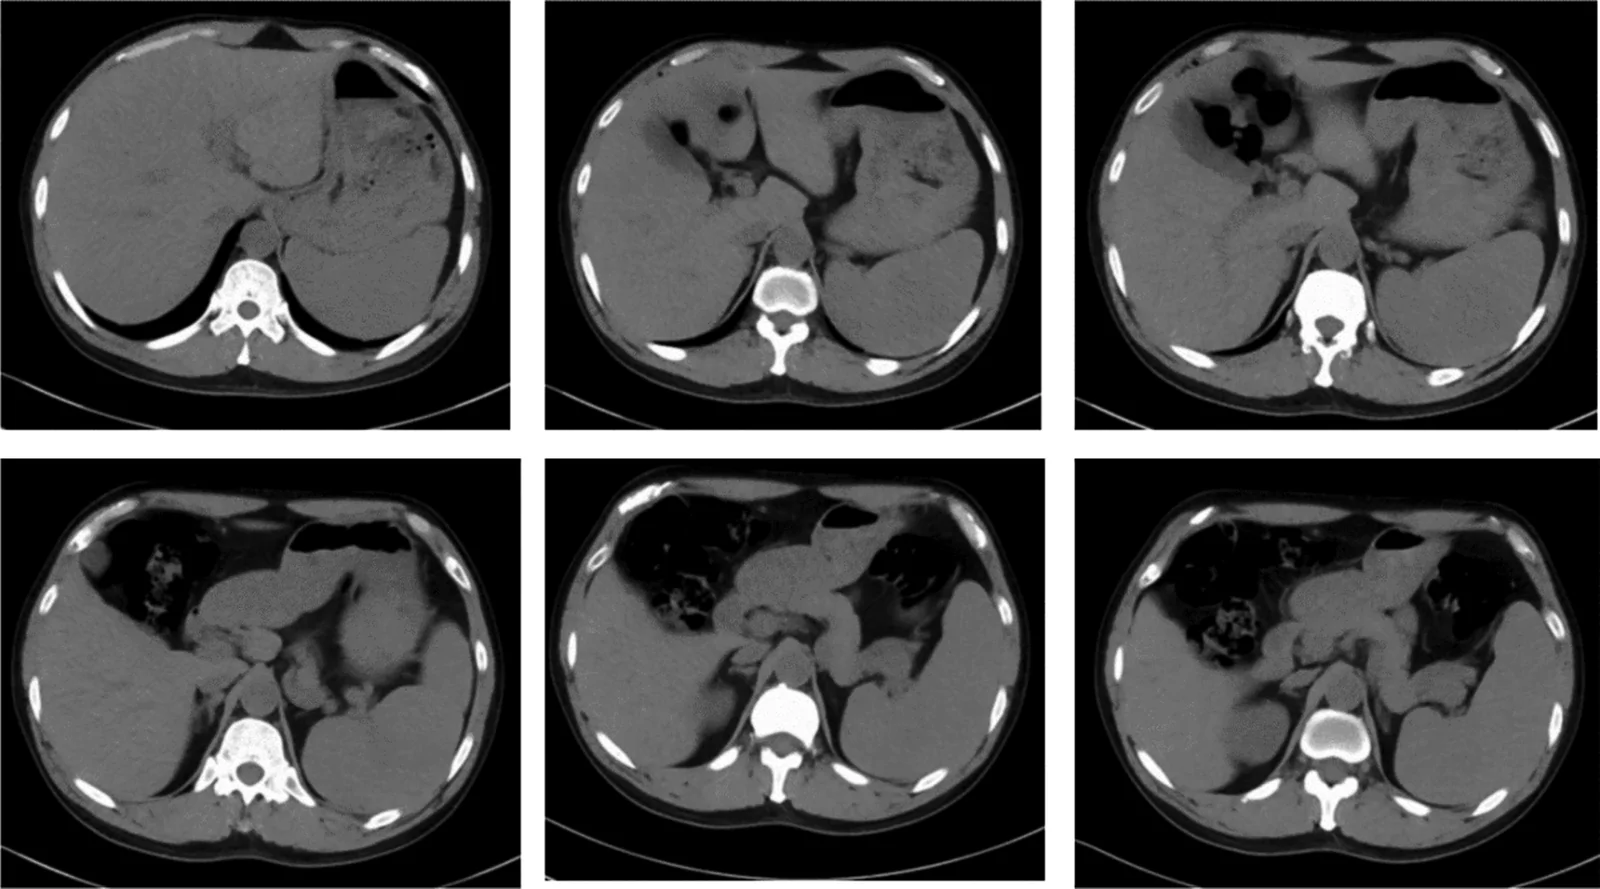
Chest CT incidentally shows splenomegaly. CT = computed tomography.
